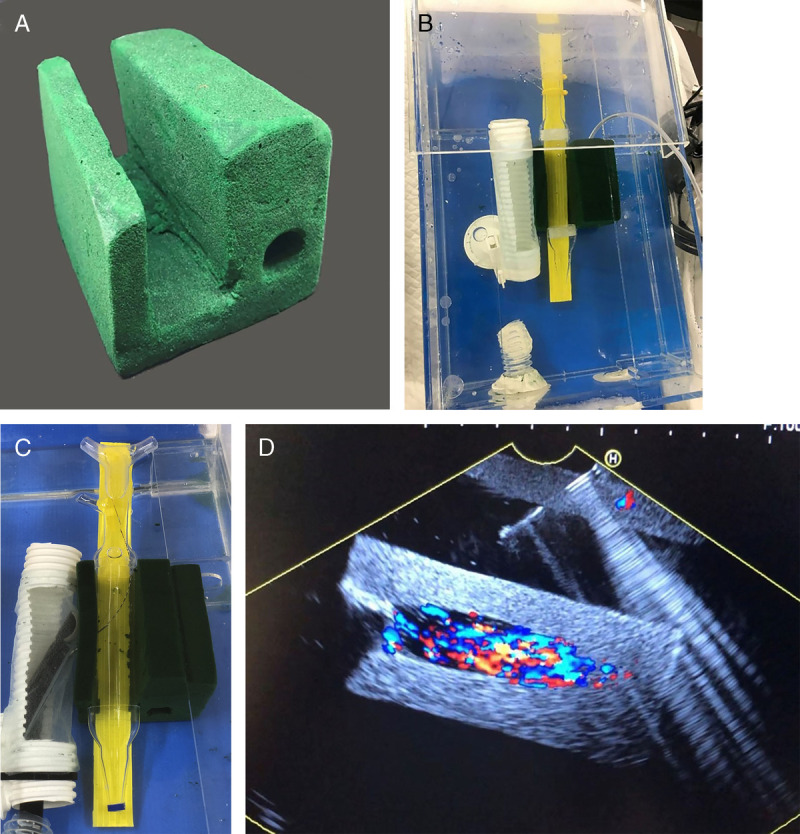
EUS-CDS model using a floral foam. The floral foam is shaped into portoduodenal soft tissue (A). A transparent plastic tube to represent the common bile duct is placed in the center along with the tunnel that represents the portal vein (B). During puncture, the instrument could be seen through the plastic tube (C), and Doppler flow could be created by pumping water through the tunnel (D). CDS: Choledochoduodenostomy.
